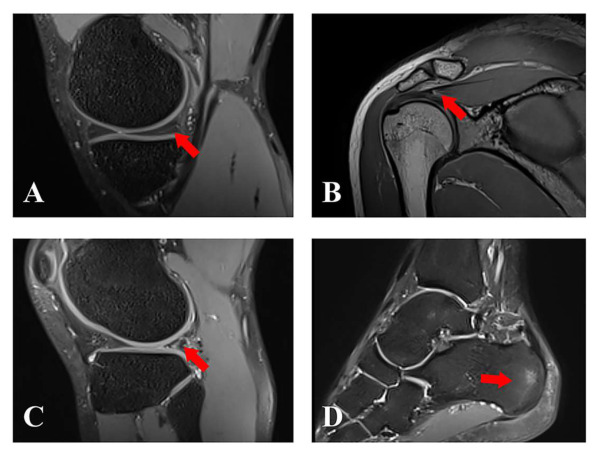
Representative MRI findings of asymptomatic joint abnormalities in collegiate athletes. (A) Sagittal PD-FS image of the right knee in a 21-year-old male badminton player: posterior horn of the medial meniscus degeneration, Grade I (arrow). (B) Sagittal T1-weighted SPACE image of the right shoulder in a 20-year-old female badminton player: supraspinatus tendinopathy, Grade II (arrow). (C) Sagittal PD-FS image of the right knee in a 21-year-old male soccer player: posterior horn of the lateral meniscal lesion, Grade II (arrow). (D) Sagittal PD-FS image of the left ankle in a 19-year-old male badminton player: calcaneal bone marrow edema (arrow).
